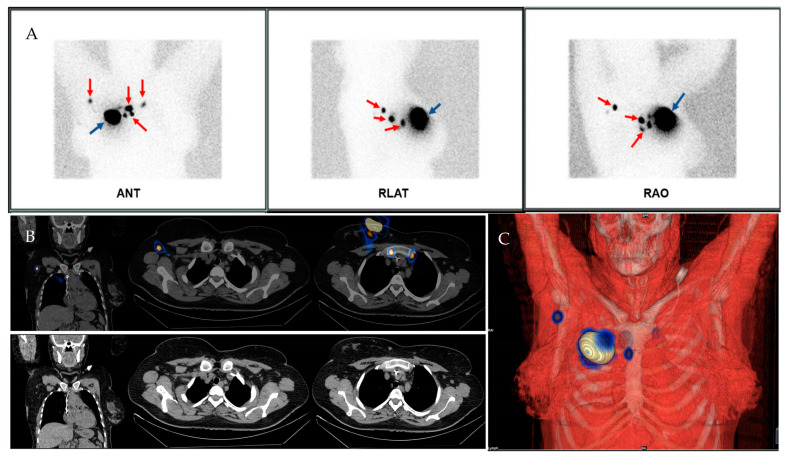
Female patient aged 45 years old diagnosed with Stage II invasive ductal carcinoma of the breast. (A) Planar lymphoscintigraphy showed multiple SLNs (red arrows) localised in different lymph node levels and overlapping the injection site (blue arrow). (B) For more precise localisation of the SLNs, the patients underwent SPECT/CT examination that revealed lymphatic drainage originating from the peritumoural region into the right axillary region (involving 1 SLN) and the bilateral internal mammary regions (involving two nodes on the right side and one on the left side). Figure (C) shows a 3D rendering of the SLNs positions.
